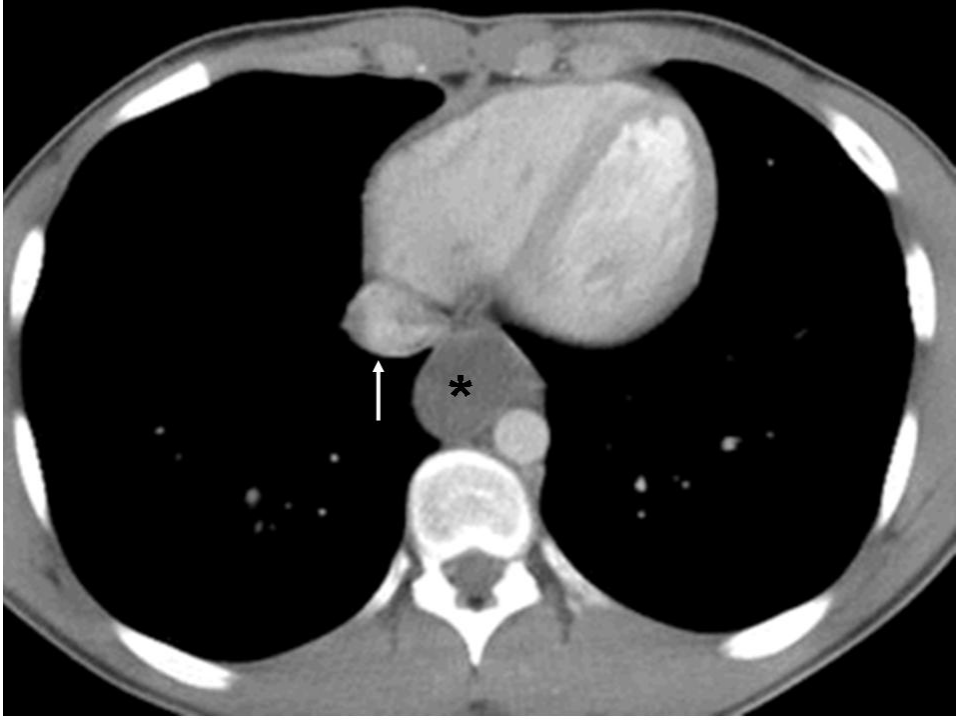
Contrast enhanced CT on a 45 years old male show a low density mass (asterisk) located posterior to the heart and anterior and to the right of the descending thoracic aorta. Oesophagus is not separately identified from the mass. Density of the mass was measured to be 7HU suggesting that it is cystic in nature. Differential considerations of cystic mass in this location are bronchogenic cyst and oesophageal duplication cyst. Arrow points to inferior vena cava as it enters right atrium
